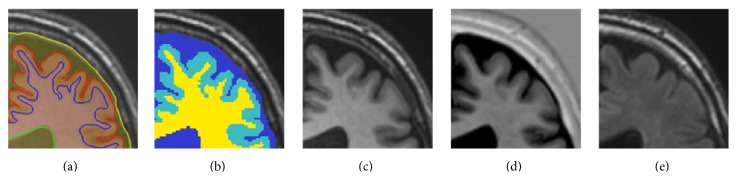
Example of the manually drawn contours (a), the resulting hard segmentation map (GM: light blue, WM: yellow, and CSF: dark blue) that is used as the reference standard (b), the T1-weighted scan (c), the T1-weighted inversion recovery (IR) scan (d), and the T2-weighted fluid attenuated inversion recovery (FLAIR) scan (e).
